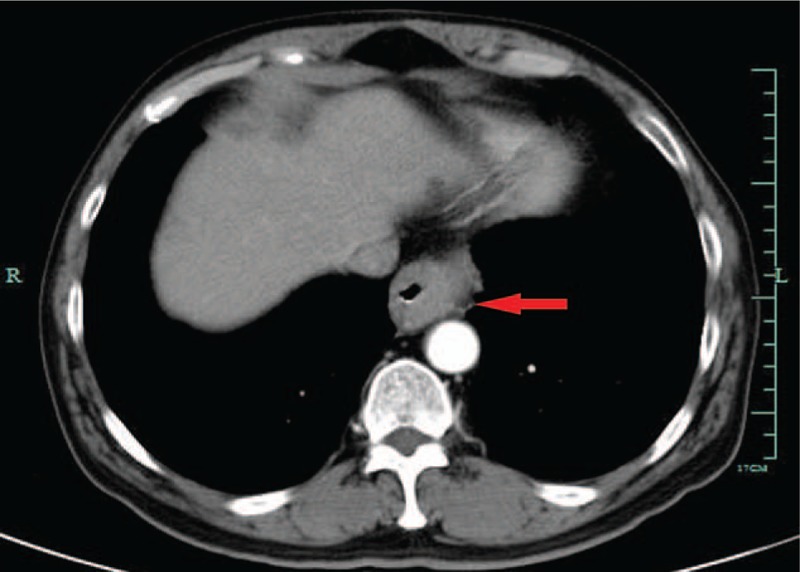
The tumor formed a luminal stenosis and was deemed to be resectable after a CT scan (arrow). CT = computed tomography.
